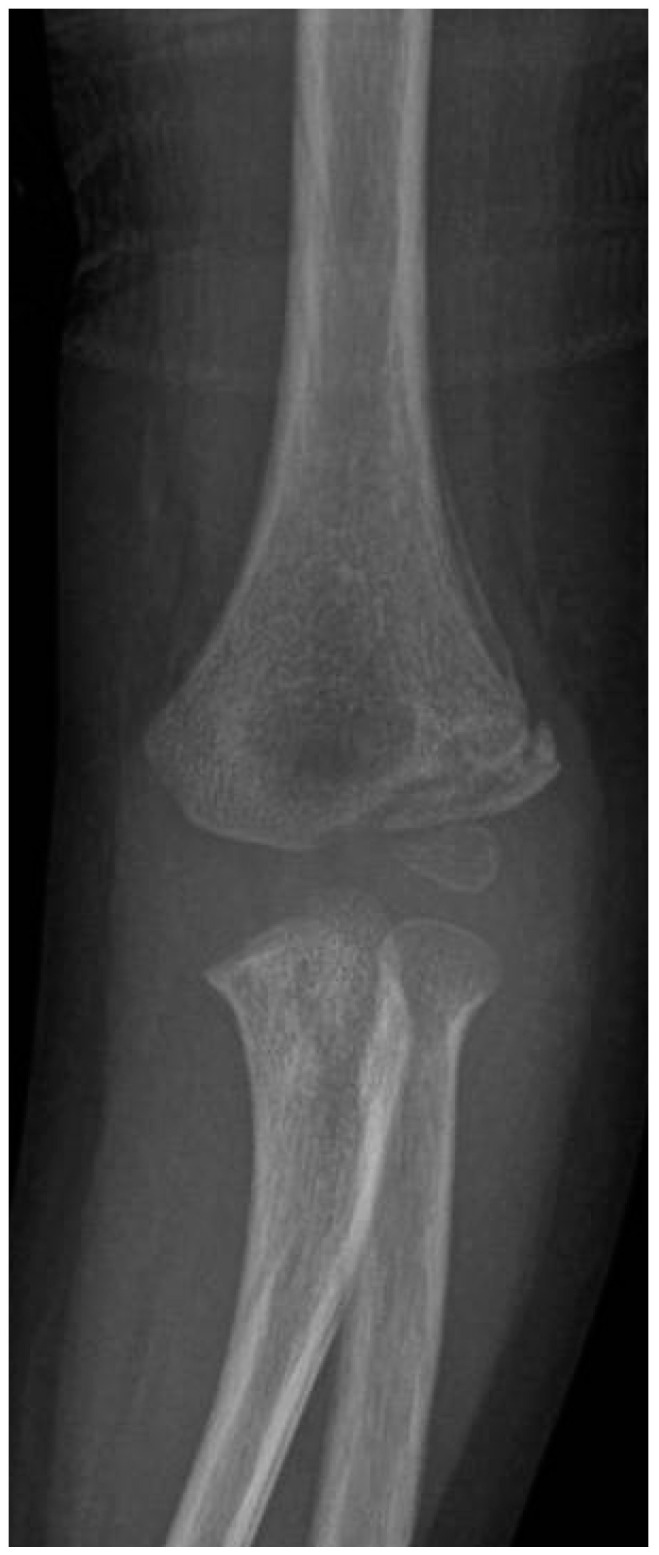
Postoperative X-ray after 30 days of immobilization with long arm cast (anteroposterior view).
